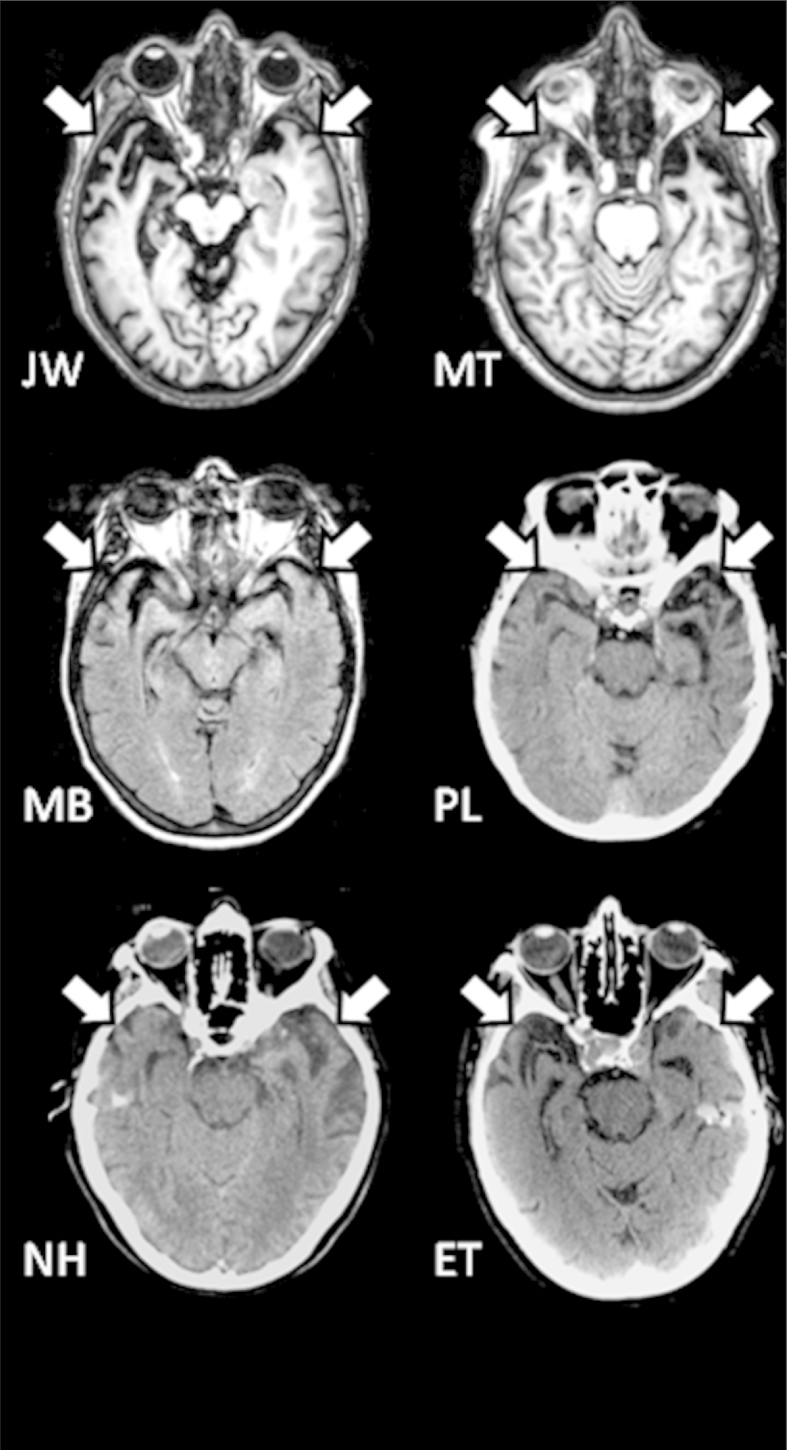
Structural imaging. Structural MR or CT images for patients, indicating anterior temporal atrophy in each case. No images were available for P.W., though an MR scan report confirmed that this patient also had anterior temporal damage.
